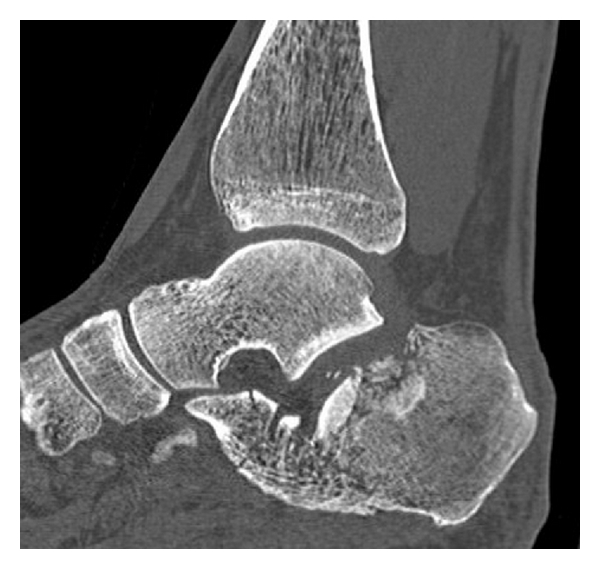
Initial sagittal computed tomography scan of the fractured calcaneus.
